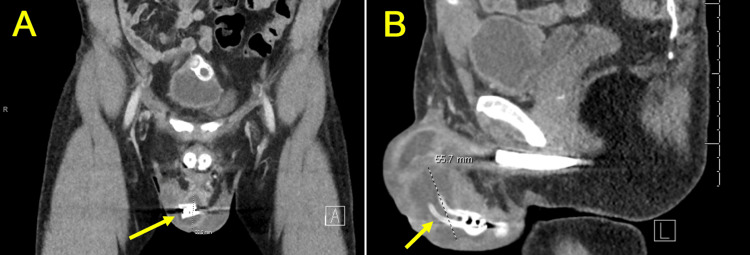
Pelvic CT scan with contrast (A) Coronal view showing an enhanced amorphous hypodensity surrounding the penile implant reservoir, potentially indicating an abscess (arrow) and (B) Sagittal view highlighting a 5.6 cm hypodense area in the mid-scrotum adjacent to the penile implant, with implant tubing passing through it (arrow).
